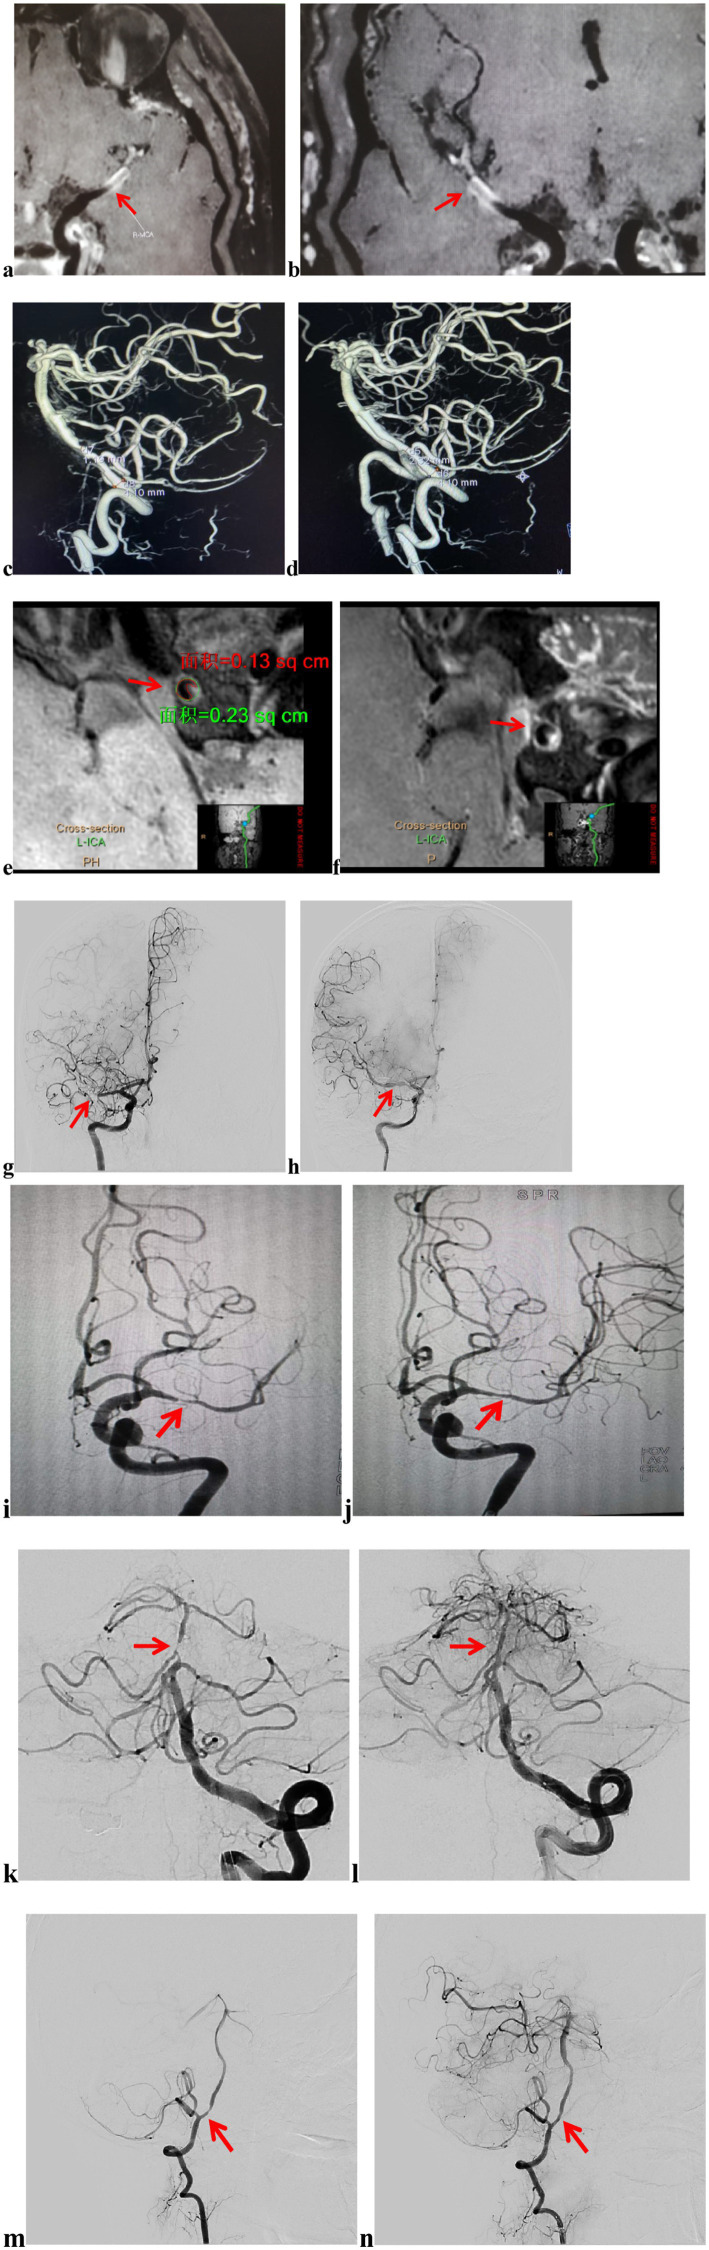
Imaging data and endovascular procedure. Preoperative VW-HRMRI showed that the lumen of M1 of the right MCA was almost occluded, but a portion of the true lumen of the vessel wall remained, making it a candidate for angioplasty and stenting (a,b). The stenosis rate = 1-(Ds/Dn), where Ds was the diameter of the artery where the stenosis was most obvious and Dn was the diameter of the proximal normal artery. The stenosis rate of the left VA was 70.98% before intracranial stenting (c), 43.41% after intracranial stenting with the Neuroform Atlas Stent (d); plaque burden was measured at the site of maximal stenosis, calculated using the formula: (outer area-lumen area)/outer area (e); the contrast enhancement ratio was measured at the site of maximum plaque enhancement, normalizing the SI by using nearby gray matter (e, post-contrast SI; f, pre-contrast SI); preoperative DSA showed that the lumen of M1 of the right MCA was nearly occluded, and the distal branches were not visualized (g); after angioplasty, a Neuroform Atlas Stent was successfully implanted in the M1 of the right MCA, and follow-up angiography 10 min later showed good blood flow (h); preoperative DSA showed that the lumen of M1 of the left MCA was severe stenosis, and the distal branches were sparse (i); after angioplasty, a Neuroform Atlas Stent was successfully implanted in the M1 of the left MCA, and follow-up angiography 10 min later showed good blood flow (j); preoperative DSA showed that the lumen of BA was severe stenosis, and the distal branches were sparse (k); after angioplasty, a Neuroform Atlas Stent was successfully implanted in the BA, and follow-up angiography 10 min later showed good blood flow (l). Preoperative DSA showed that the lumen of the left VA was severe stenosis, and the distal branches were sparse (m). After angioplasty, a Neuroform Atlas Stent was successfully implanted in the V4 of the left VA, and follow-up angiography 10 min later showed good blood flow (n). VW-HRMRI, vascular wall high-resolution magnetic resonance imaging; MCA, middle cerebral artery; VA, vertebral artery; SI: signal intensity; DSA, digital subtraction angiography; BA, basilar artery.
